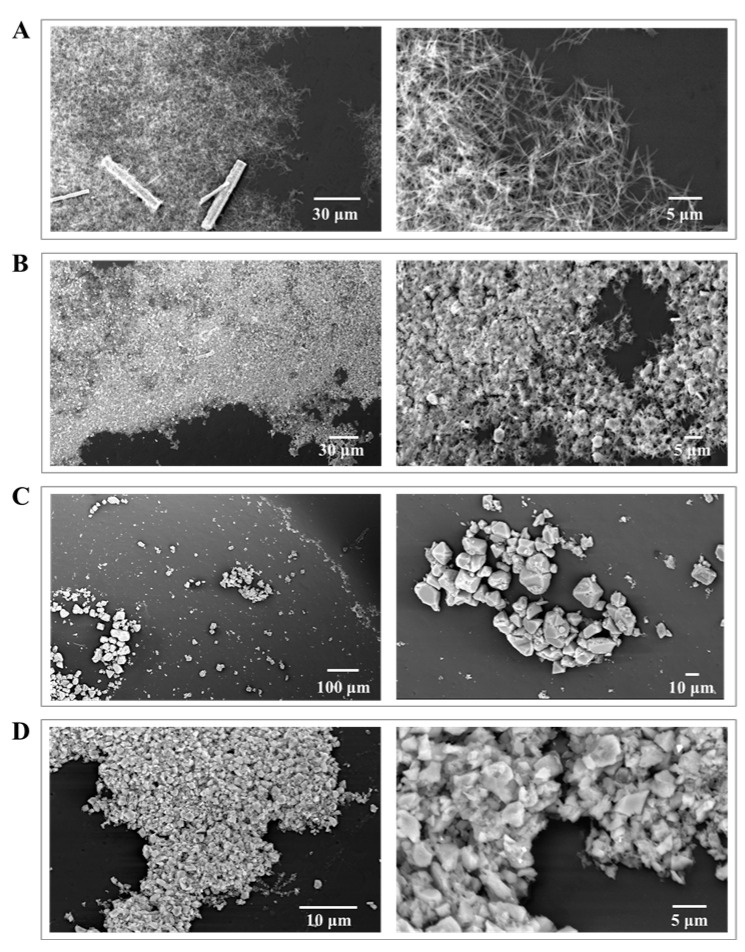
SEM images of HKUST-1 coatings on 96-well crystallization plates obtained using different solvents and treatments: water solvent and ultrasound (A), water solvent and high pressure (B), alcohol solvent and ultrasound (C), and alcohol solvent and high pressure (D).
